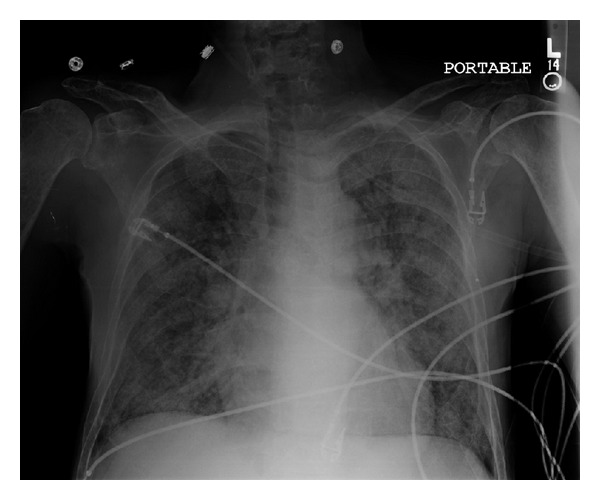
Chest X-ray AP view showing reticulonodular infiltrates and microcalcifications.
